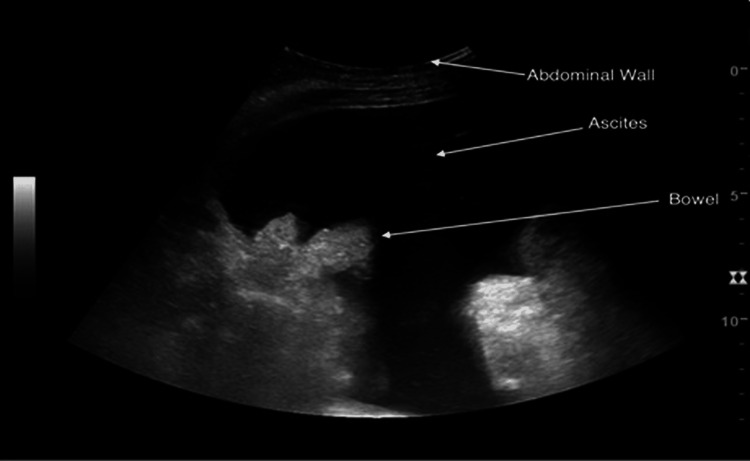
An ultrasound image showing moderate ascites Abdominal ultrasound, performed with a curvilinear probe (LOGIQ E9 system; GE HealthCare, Wauwatosa, WI, USA), demonstrates a large anechoic (dark) fluid collection within the peritoneal cavity, consistent with ascites. The abdominal wall is visualized superficially at the top, while echogenic bowel loops are seen floating within the ascitic fluid. This image highlights the characteristic sonographic appearance of ascites.
